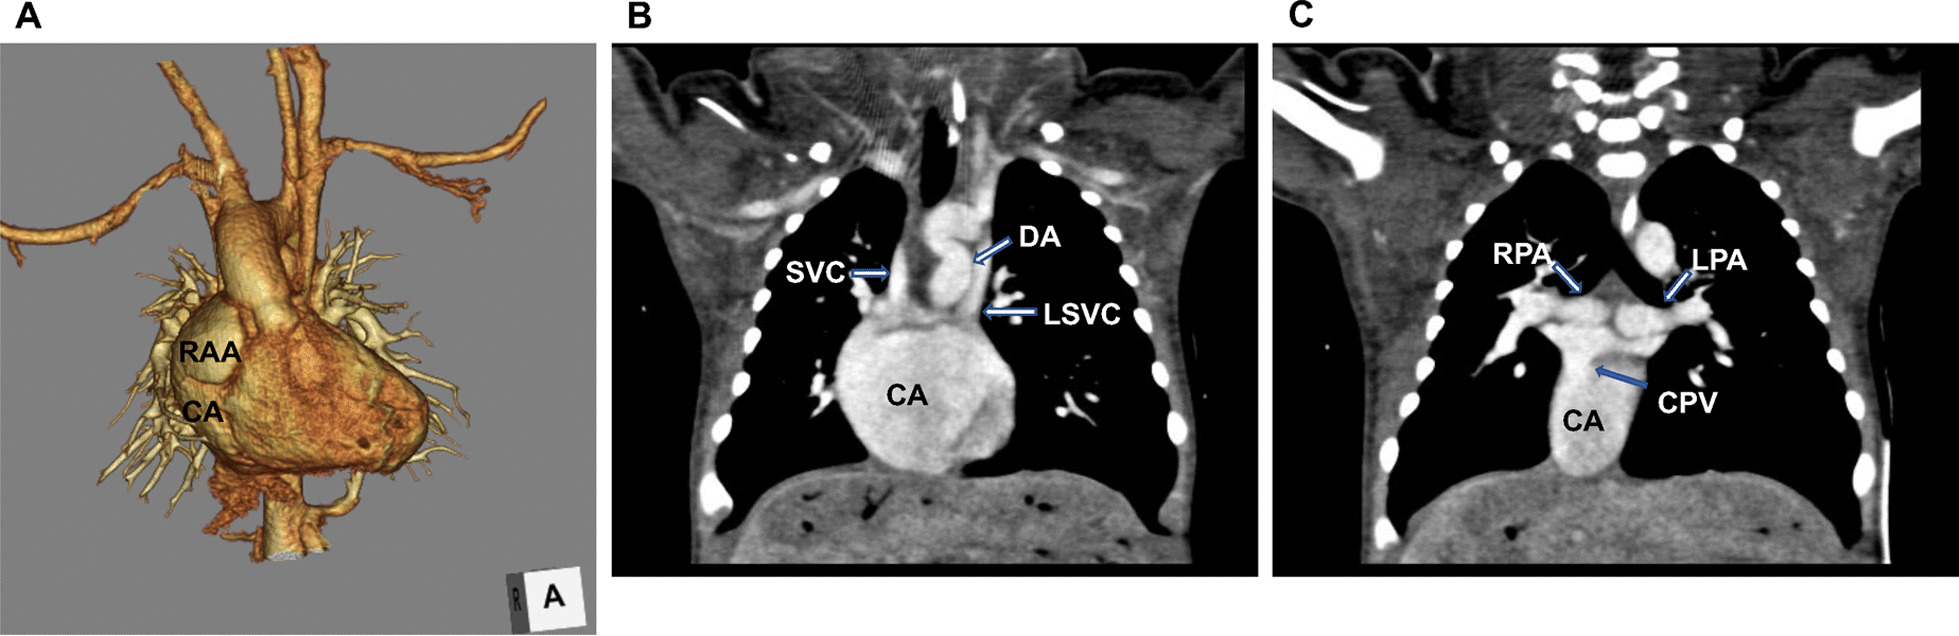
Preoperative contrast-enhanced computed tomography (CT) findings. A 3DCT image showing the right-sided juxtaposition of the atrial appendage overhanging the common atrium. B Coronal CT image showing the bilateral superior vena cava connected to the ceiling of the common atrium; large DA. C Coronal CT image showing CPV connected to the right-side common atrium. CA, common atrium; RAA, right atrial appendage; DA, ductus arteriosus; SVC, superior vena cava; LSVC, superior vena cava; RPA, right pulmonary artery; LPA left pulmonary artery; CPV, common pulmonary vein
